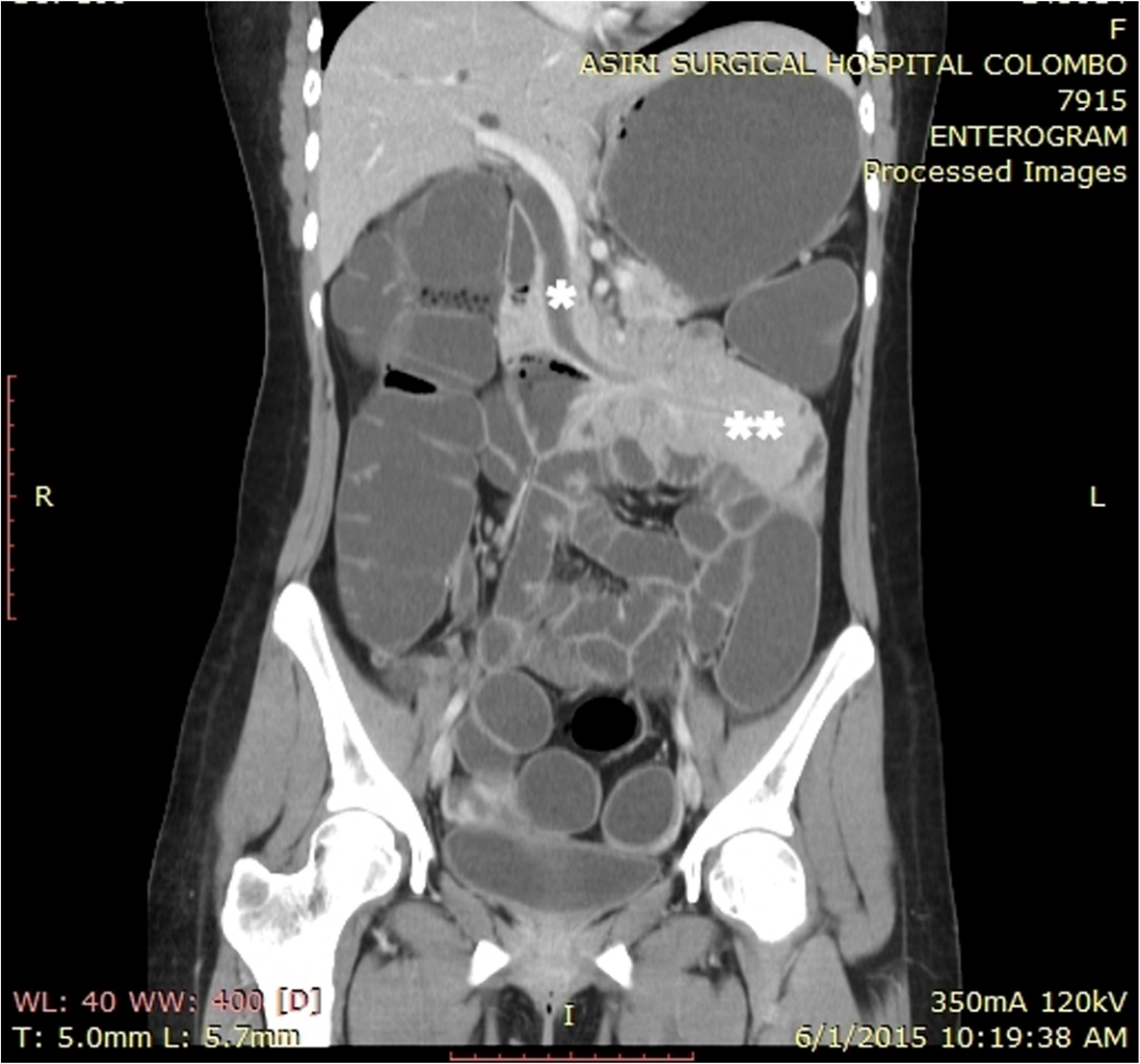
A coronal reconstruction of computed tomography enterogram showing elongated and dilated common bile duct with the lower end on the left side of the midline (*) and the intussusception with the intussusceptum in the proximal jejunum ()
